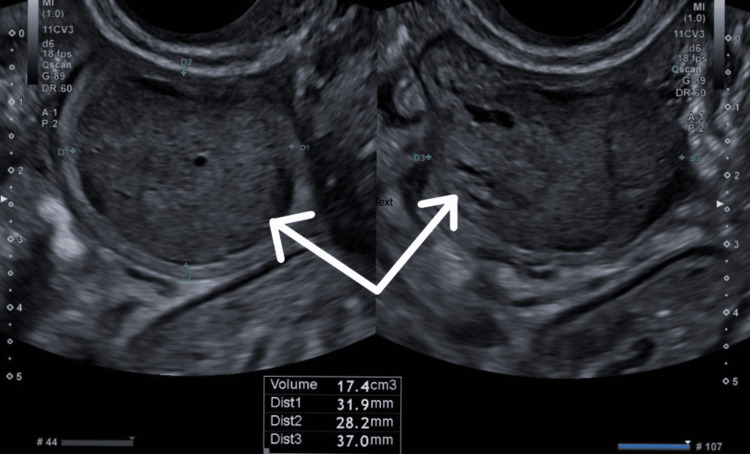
Transvaginal ultrasound imaging showing a left ovarian mass
